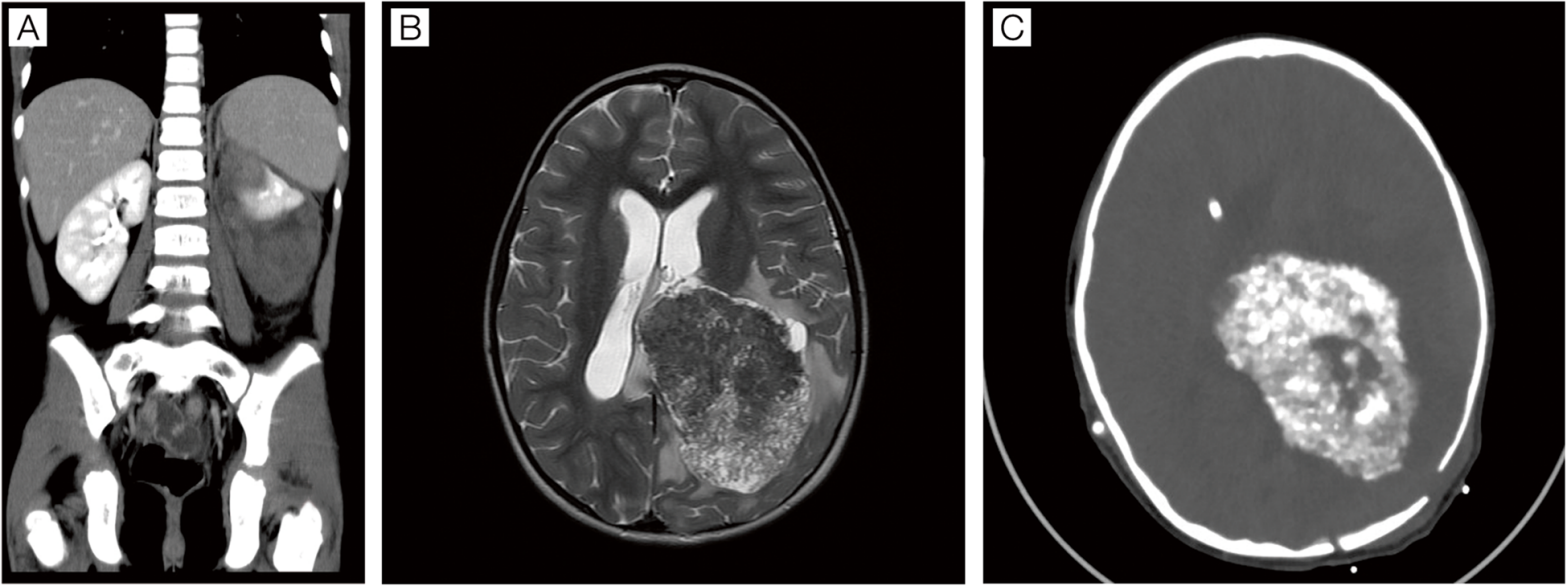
Radiological studies. Panel a Intravenous contrast-enhanced abdominal CT, coronal view: a solid, heterogeneous tumor in the upper pole of the left kidney is identified (upper, thin arrow), with an adjacent subcapsular perirenal hematoma (lower, thick arrow). The actual diameters were 36 mm × 28 mm × 27 mm in anteroposterior, transverse, and craniocaudal planes. Panel b Cerebral MRI, T2-weighted sequence, axial view. A solid supratentorial intraventricular tumor with left parietal lobe extension is shown. Cystic areas in the basal peripheral and medial aspects are identified .The tumor causes localized ventriculomegaly, vasogenic edema and midline shift. The actual diameters (tumor and cysts) were 81 × 59 × 75 mm in the anteroposterior, transverse, and craniocaudal planes. Panel c Non-enhanced Brain CT scan after shunt insertion. Axial reconstruction. A left parietal tumor with extensive calcification is shown. Ventricular catheter tip is in the right frontal ventricular horn
